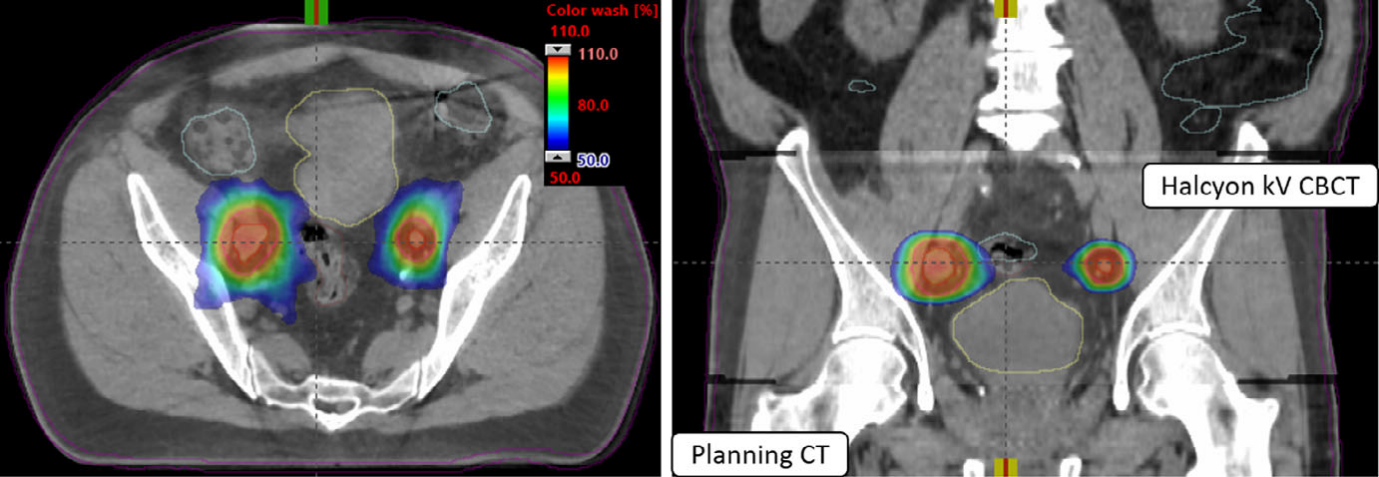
Axial and coronal views of Halcyon kV‐CBCT images (see inset in the coronal view) co‐registered with planning CT images used for image‐guided single‐isocenter (see, cross‐hair) VMAT‐SBRT treatment to both lesions on Halcyon is shown. In addition to anatomical landmarks, the planned dose colorwash (50%–110% isodose cloud) is overlaid for this treatment to demonstrate the delivery accuracy. Halcyon kV‐CBCT images were acquired in the treatment position in free breathing and rigid‐registration was performed via automatic image‐registration mode on Halcyon followed by manually fine‐tuning the registration for better alignment of both nodes via soft‐tissue alignment.
